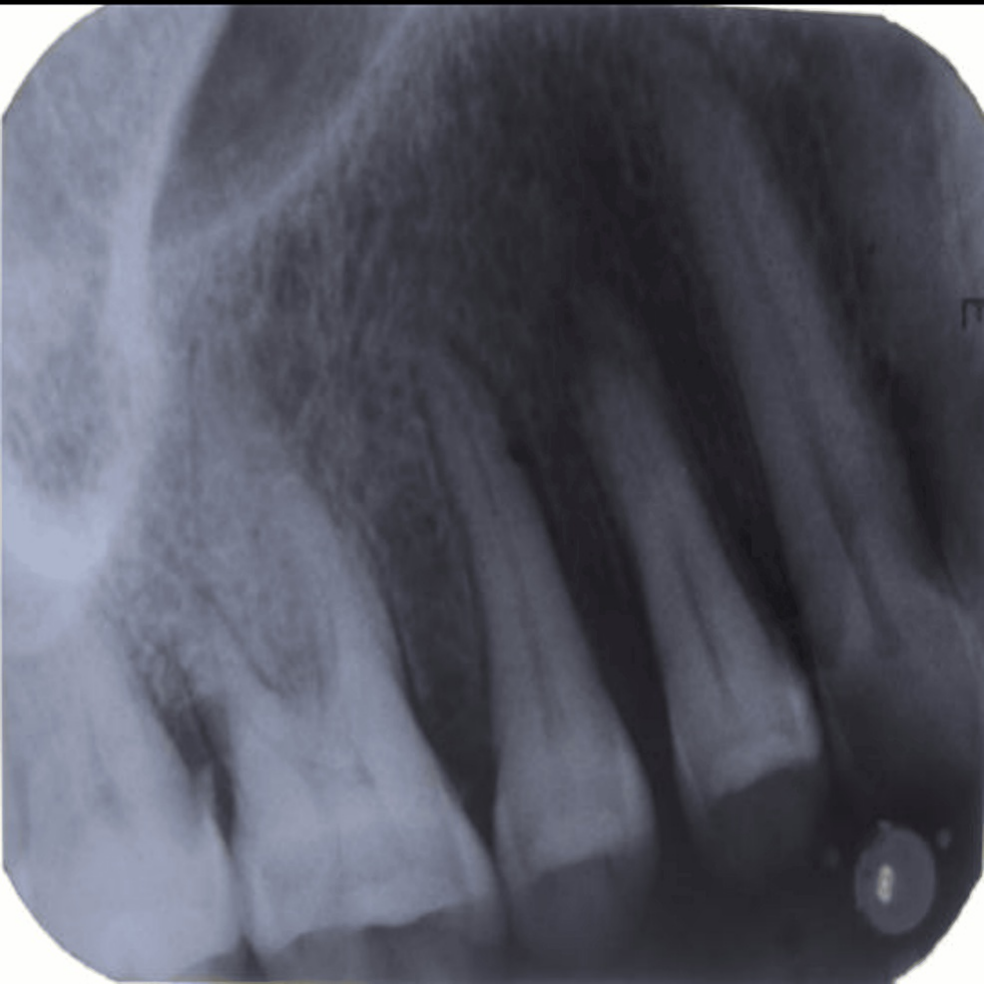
Radiographic changes of the pyogenic granuloma with respect to the affected area
